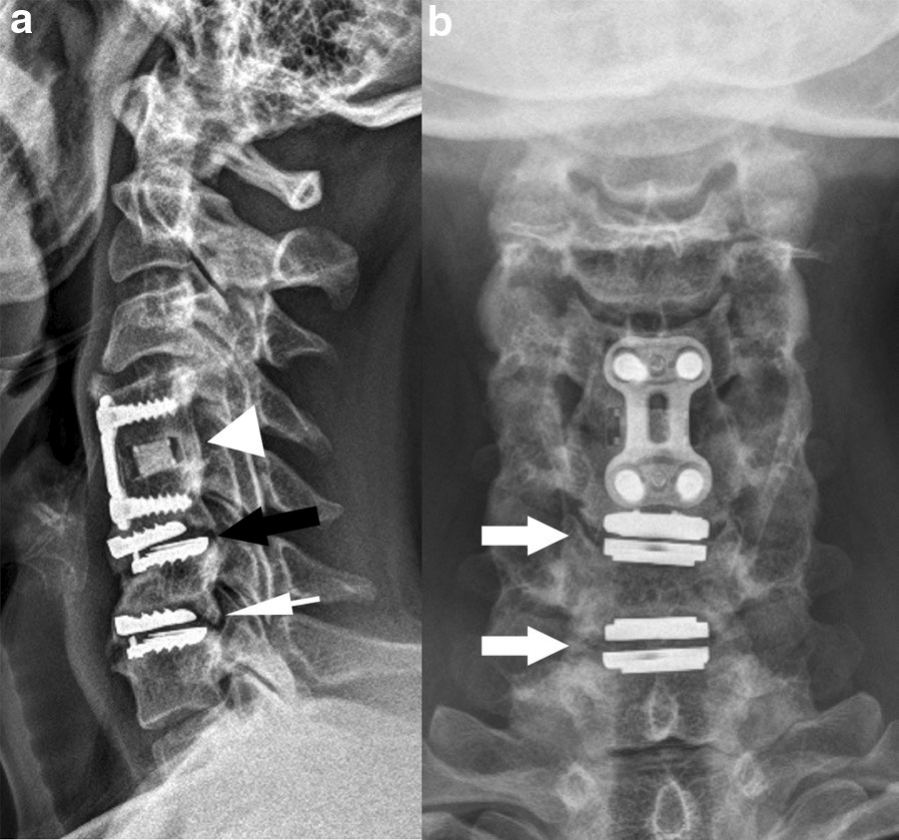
Total disc replacement (or disc arthroplasty). Lateral (a) and anteroposterior (b) radiographs demonstrate disc arthroplasty at C5-6 and C6-7 levels. There is also an anterior fusion/stabilization hardware with plates, screws, and an interbody spacer (arrowhead) at the C4-5 level. Disc prostheses (arrows) are composed of a core sandwiched between two metal plates that attach to the vertebral bodies. Ideally, the plates must be at the center of the vertebral body and parallel to each other. In this case, there is a slight anterior dislocation of the superior plate at C5-6 level (black arrow) and optimal positioning at the C6-7 level (thin arrow)
